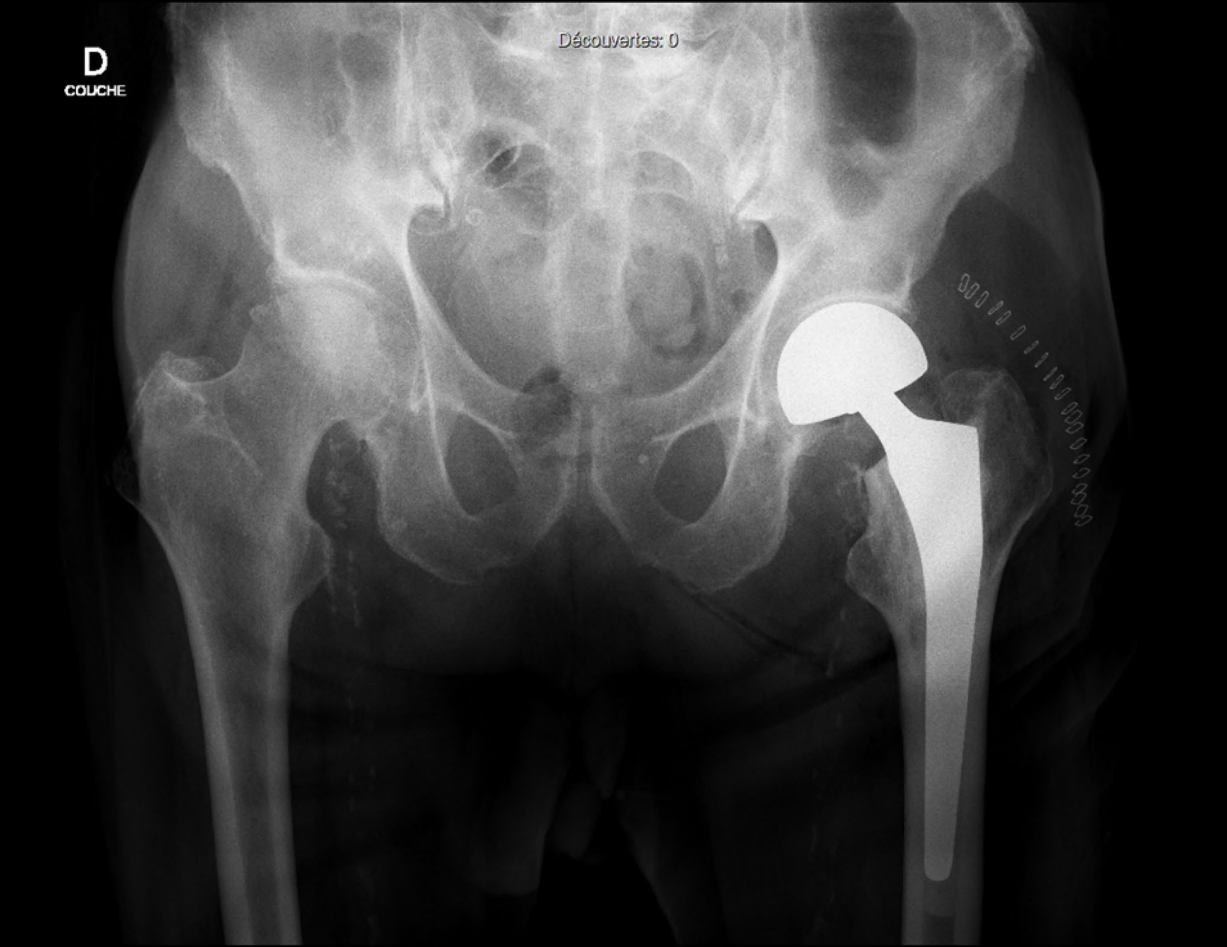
Postoperative anteroposterior pelvis radiography obtained within 24 h of surgery
